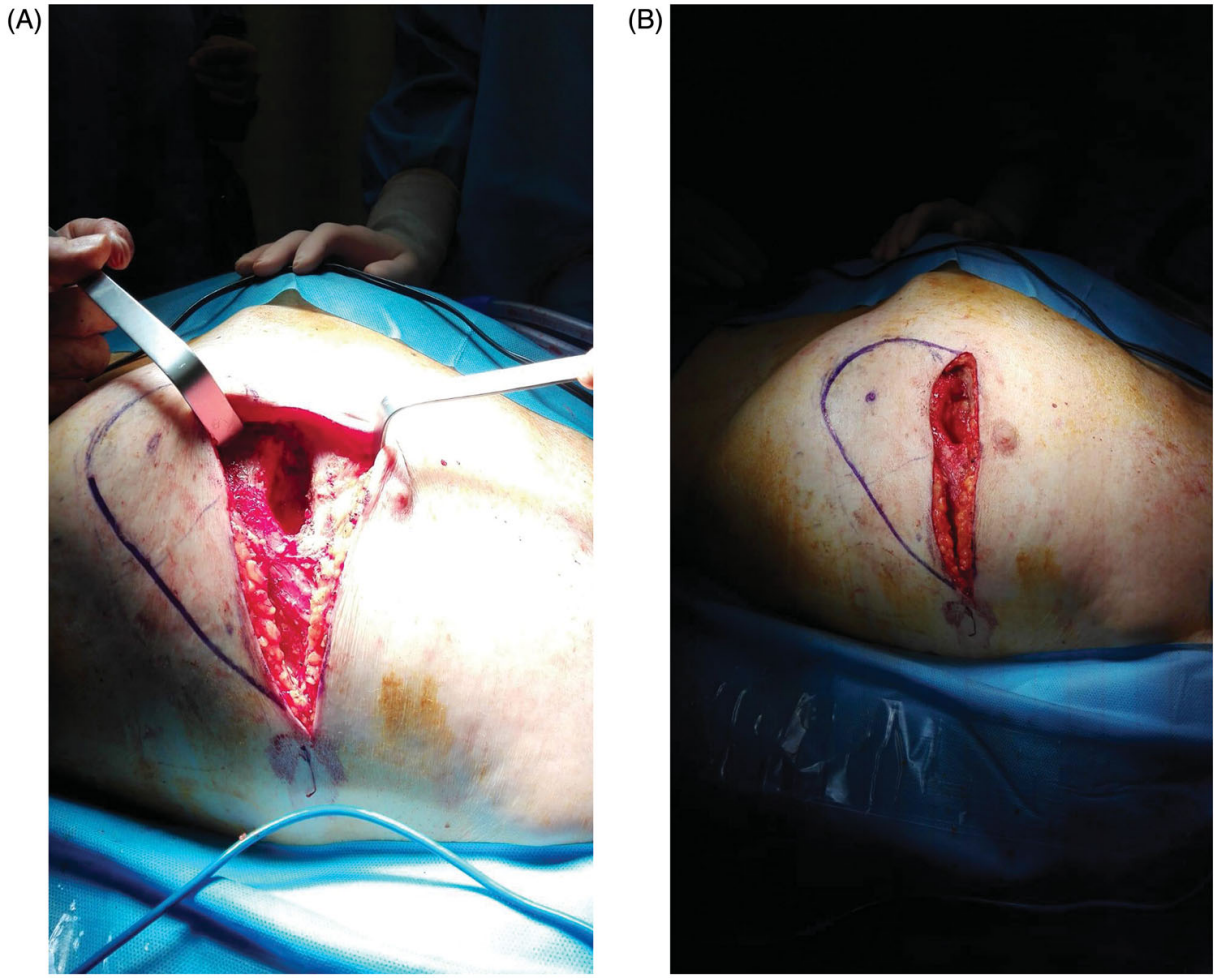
(A) The defect after debridement. (B) Flap design. The blue dot marks the perforator identified with the handled doppler probe.
